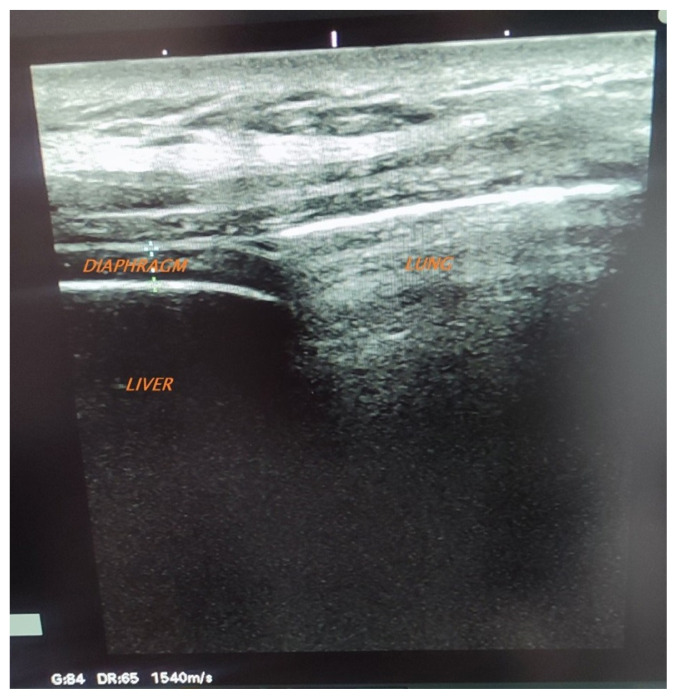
Ultrasound image of the normal right hemidiaphragm in the zone of apposition.
